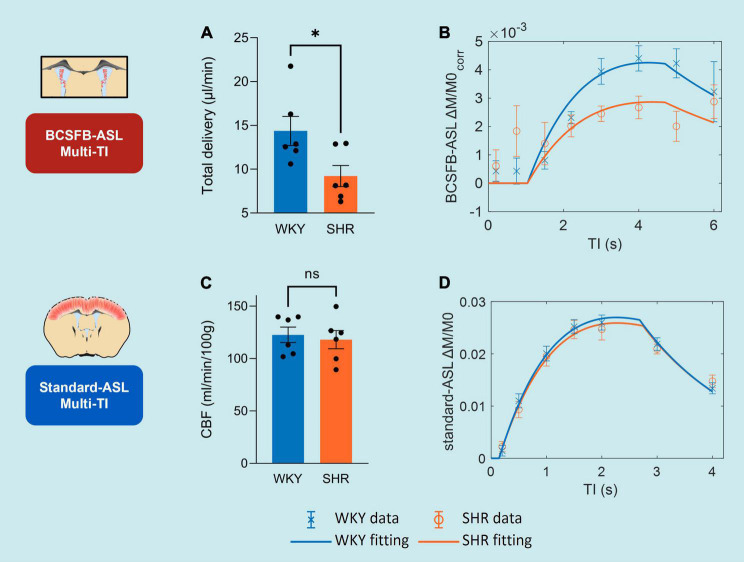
CSF homeostasis: T1_*CSF*_ and lateral ventricular volume. (A) T1_*CSF*_ values from WKY and SHR subjects, extracted from BCSFB-ASL multi-TI data. Error bars: ± SEM (*n* = 6 WKY, 6 SHR). (B) Example of multi-TI inversion recovery fitting (*n* = 1 WKY, 1 SHR). Error bars: ± stdev (20 repetitions at each TI). (C) Lateral ventricular volume values from WKY and SHR subjects, from T2-weighted anatomical images. Error bars: ± SEM (*n* = 6 WKY, 6 SHR). (D) Examples of T2-weighted anatomical images (equivalent slices) displaying ventriculomegaly (*n* = 1 WKY, 1 SHR). Asterisks: 2-tailed *t*-test significance, p* < 0.05, *p* < 0.01, p* < 0.001, and *p* < 0.0001.
